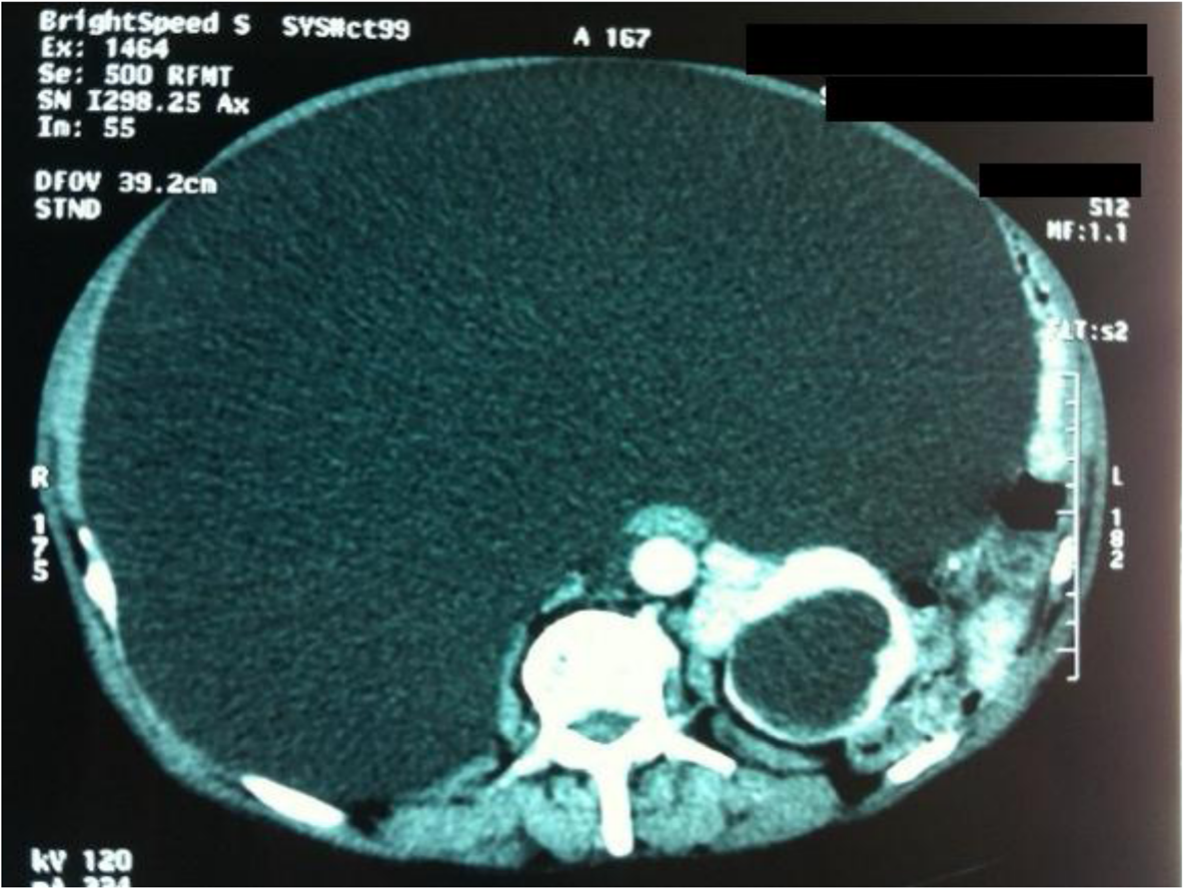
Computed tomography of abdomen revealing bilateral simple renal cysts with an exceptionally giant sized renal cysts measuring 35 × 32 × 22 cm on the right kidney.
